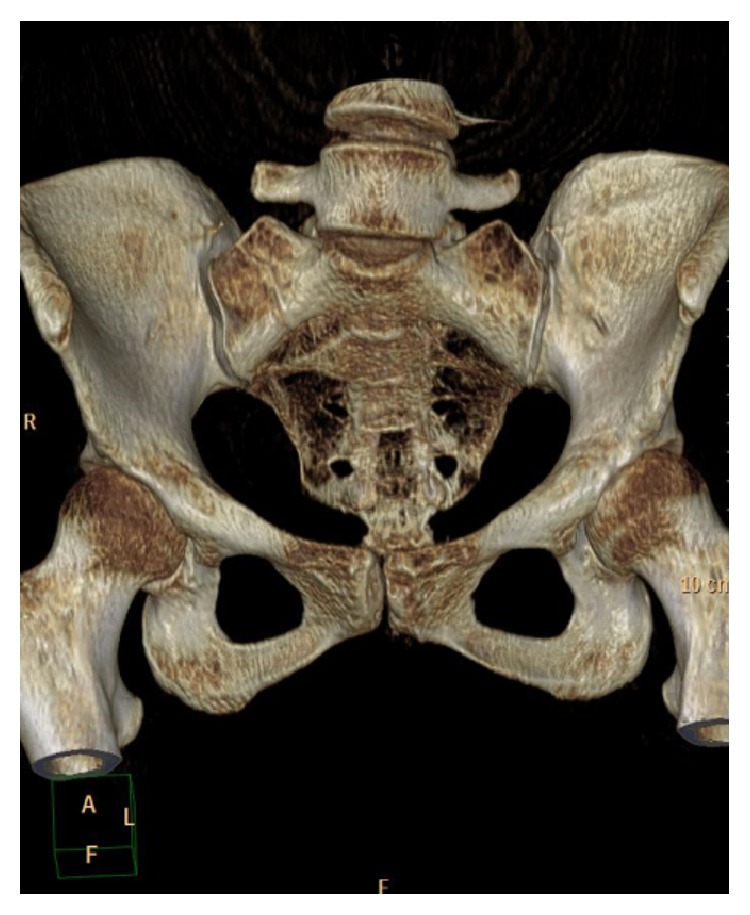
CT scan 3D reconstruction of the pelvis that showed the oblique pattern of fracture line of right sacral bone.
